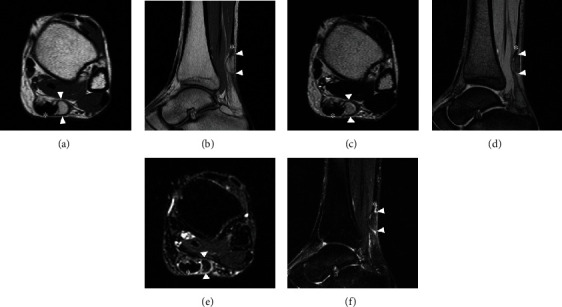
Magnetic resonance (MR) imaging at first visit. Axial (a, c, and e) and sagittal (b, d, and f) T1-weighted (T1WI, a and b), T2-weighted (T2WI, c and d), and fat-suppressed T2-weighted (T2FS, e and f) MR images.Images show a lesion (arrowhead) at the lateral aspect of the Achilles tendon (asterisk). It is isointense relative to subcutaneous fat and is surrounded by a low signal intensity area, which allows it to be distinguished from adjacent adipose tissues. The continuity of the Achilles tendon was maintained, and no tendon injury was indicated.
